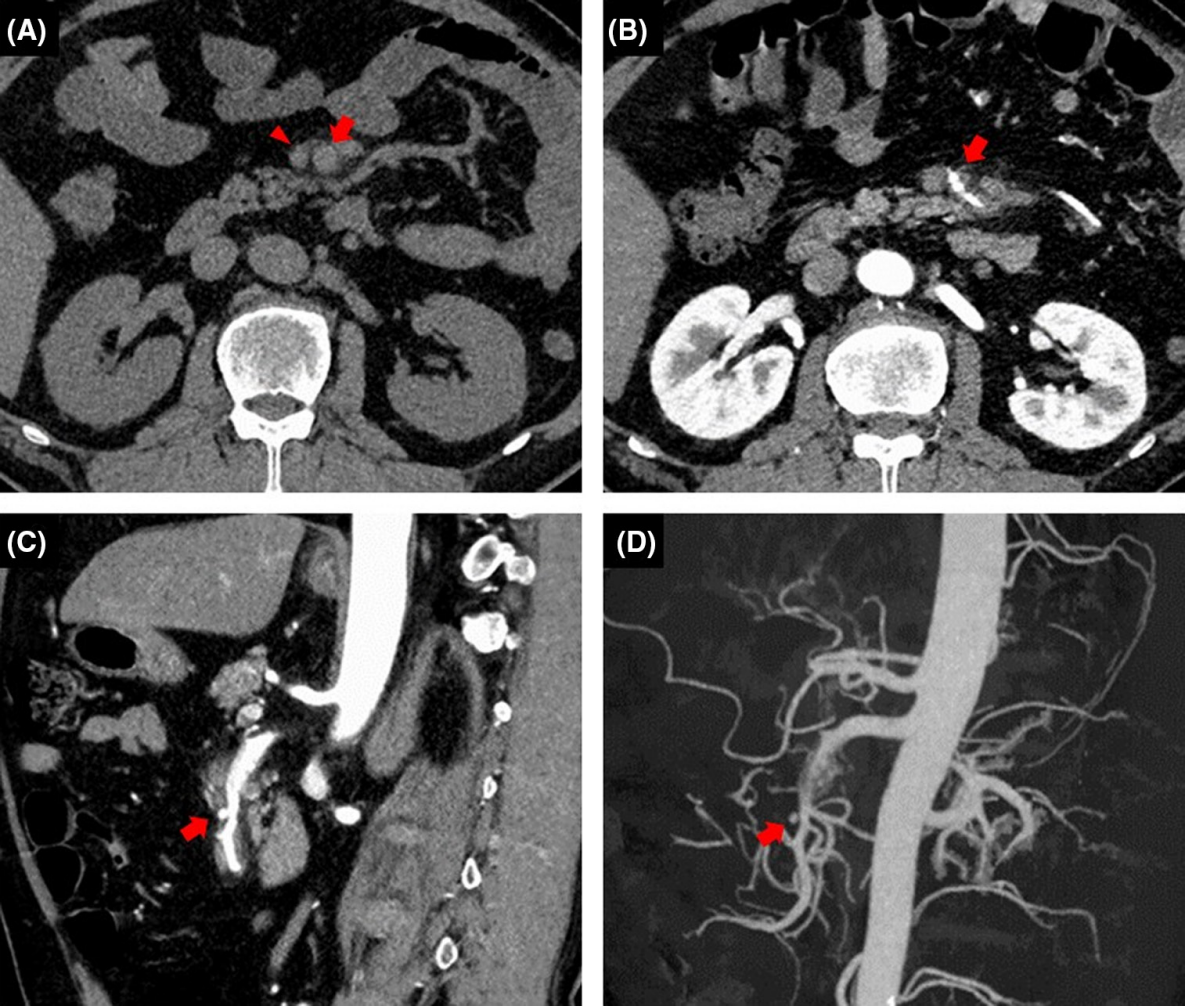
Findings of abdominal computed tomography (CT) and CT angiography on admission. (A) Axial imaging without contrast enhancement. (B) Axial imaging with contrast enhancement. (C) Sagittal imaging with contrast enhancement. (D) Three‐dimensional abdominal CT angiography. (A) Axial abdominal CT imaging without contrast enhancement showed the dilated superior mesenteric artery (SMA) (arrow) with the relatively decreased superior mesenteric vein (SMV) (arrowhead), resulting in the so‐called “smaller SMV sign.” (B–D) Horizontal and sagittal abdominal CT imaging with contrast enhancement and three‐dimensional CT angiography showed the formation of a false lumen in the SMA (arrow). These findings indicated the presence of dissection in the SMA.
